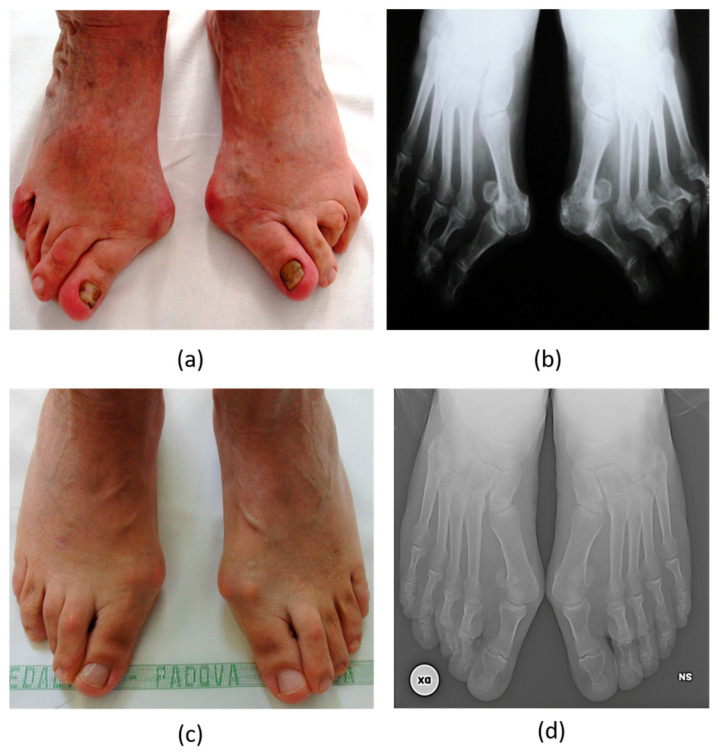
A 69-year-old woman (a,b) with evident forefoot deformities: (a) clinical image showing severe hallux valgus and lesser toe deformities including fixed claw toes and (b) weightbearing antero-posterior radiographic images showing the severe valgus of the big toe with osteoarthrosis of the metatarso-phalangeal joint, complete lateral sesamoid dislocation, and claw toes deformities of the lateral rays with dislocation of the metatarsal phalangeal joints on the left foot. A 71-year-old man (c,d) with forefoot pathologies: (c) clinical image showing moderate hallux valgus and some lesser toe deformities, including reducible claw toe deformities and (d) weightbearing antero-posterior radiographic images, showing the moderate hallux valgus without osteoarthrosis of the metatarso-phalangeal joint, sesamoid dislocations, and some claw toes deformities of the lateral rays without dislocation of the metatarsal phalangeal joints.
